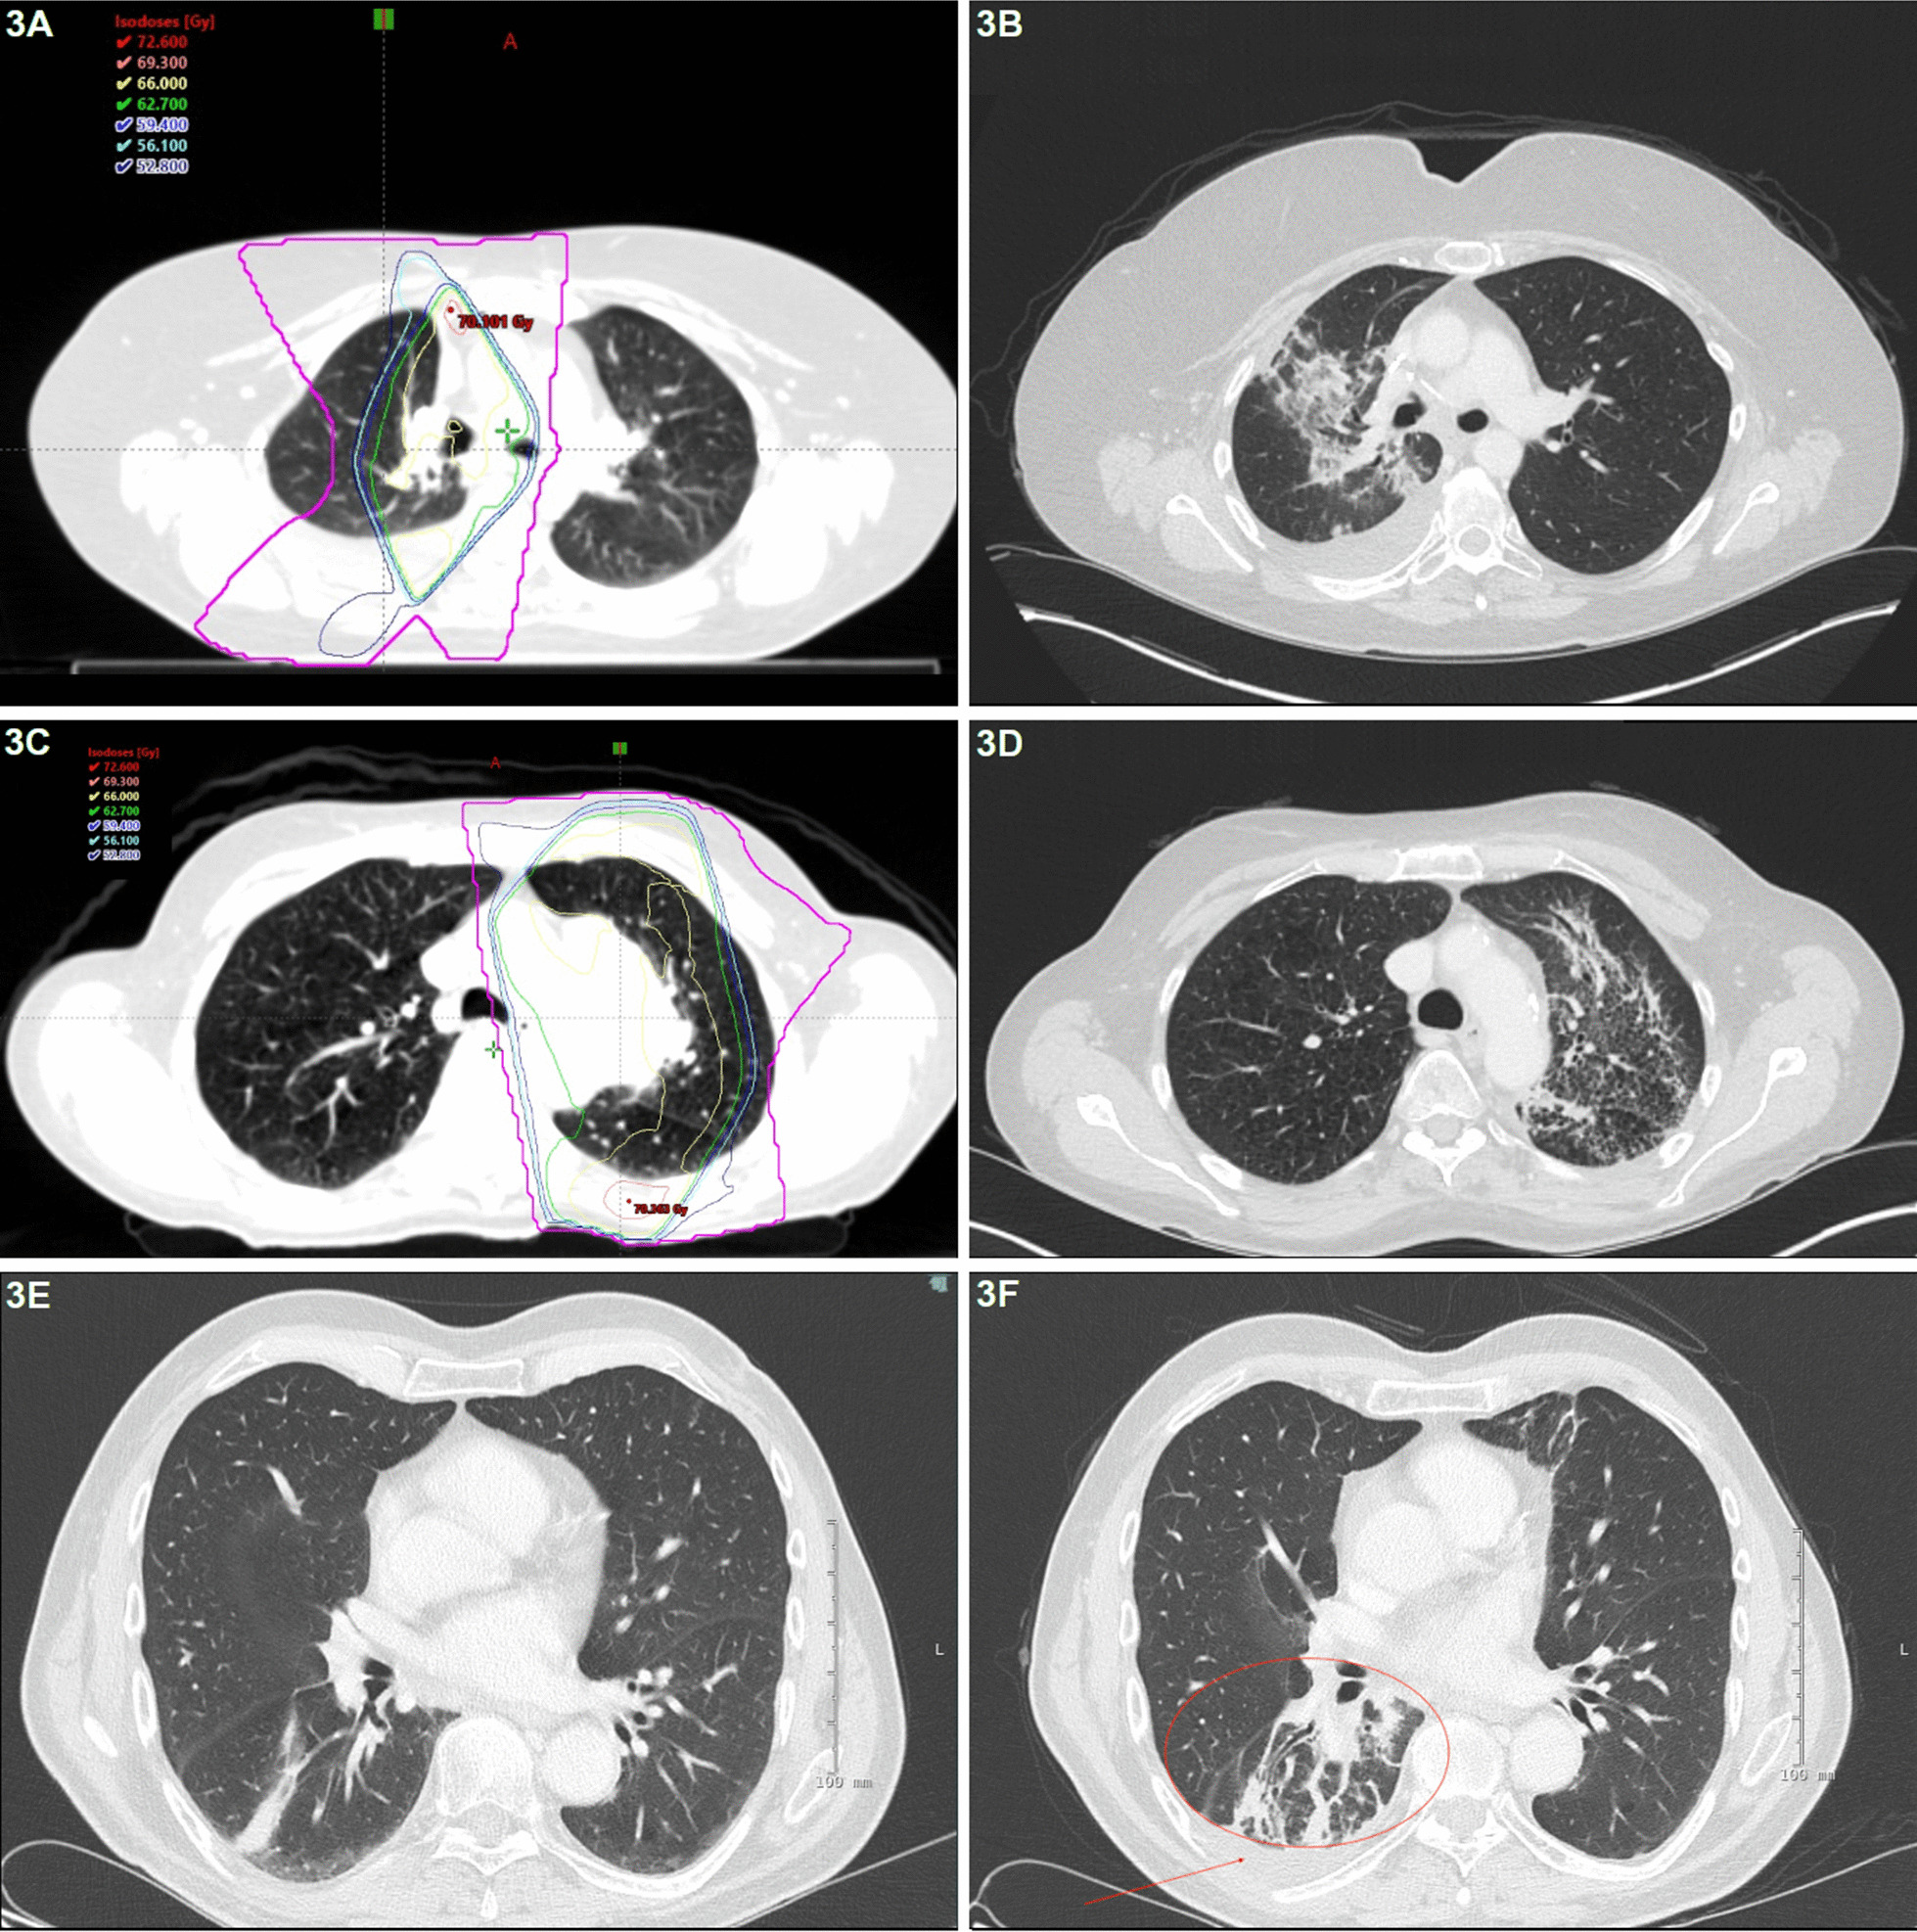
Planning computed tomography (CT) scan with relation to the physical dose distribution. Baseline CT scan before radiation therapy of patient 1 (a) and patient 2 (c), RGS grade 2 in patient 1 (b) and RGS grade 3 in patient 2 (d), escalation of RGS in patient 3 on a follow-up CT one month (e) and three months (f) after RT; *isodose legends: yellow – 66 Gy, green – 62.7 Gy, blue – 59.4 Gy, azure – 56.1 Gy, navy – 52.8, violet – 27 Gy, circle – consolidations, arrow – pleural effusion* (RGS, radiation-induced lung injury grading scale)
